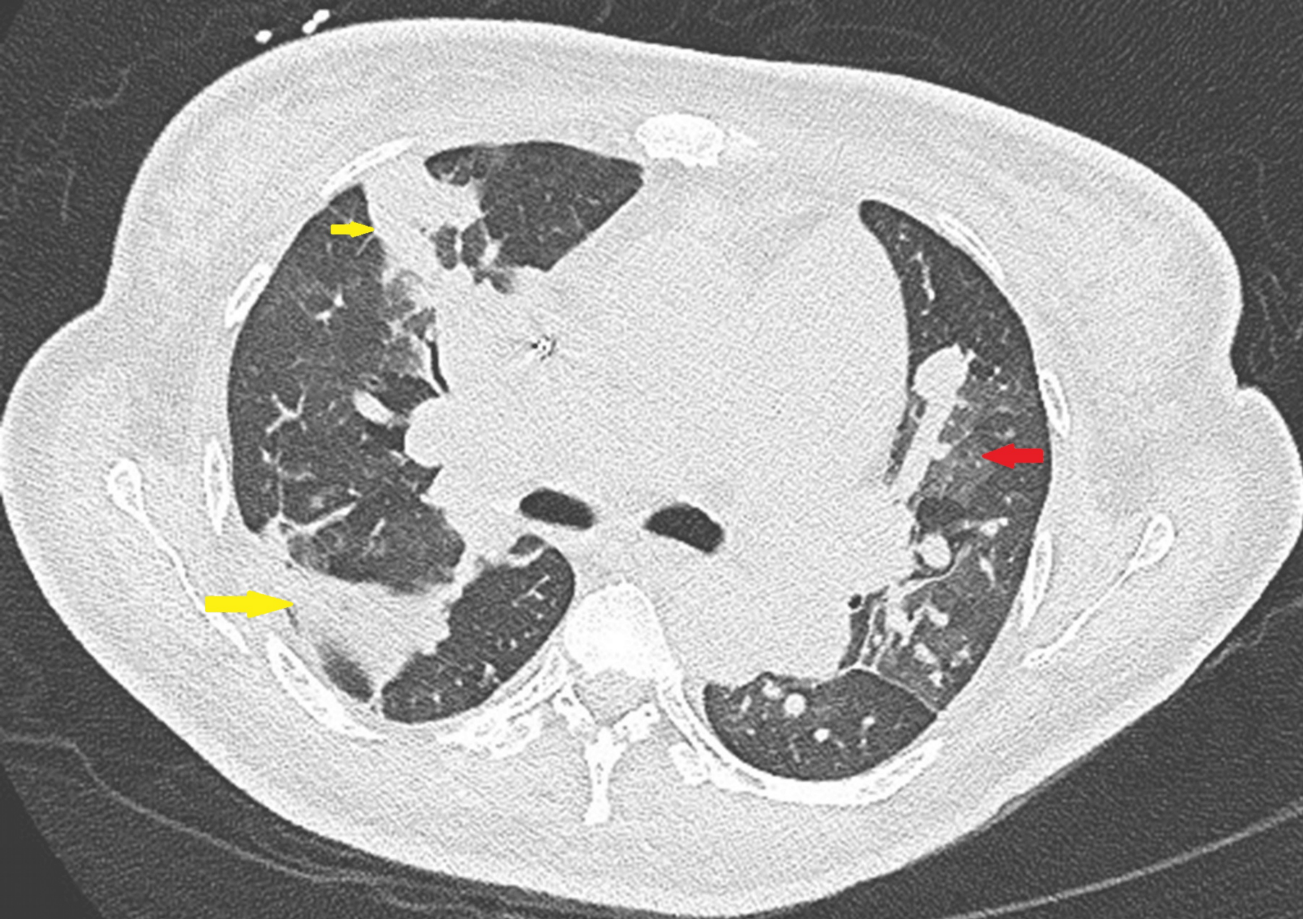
Axial CT scan showing pulmonary septic emboli (yellow arrows) and pulmonary infarction (red arrow) CT: computed tomography
